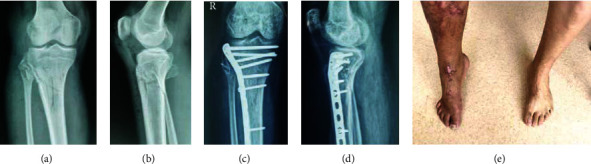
A tibial plateau fracture case with type 2 fibular column injury fixed with a steel wire. The patient showed iatrogenic peroneal nerve injury with foot drop and paresthesia postoperatively: (a, b) preoperative X-ray; (c, d) postoperative X-ray; (e) the symptom of right foot drop.
